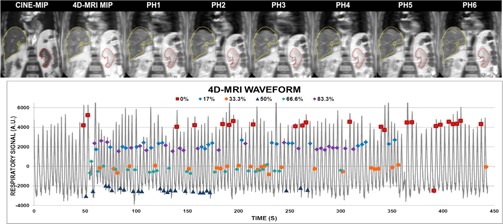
(Top) Coronal view for six‐phase 4D MRI for a liver cancer patient acquired in ~7.4 min. Contours derived from CINE‐MIP. (Bottom) 4D MRI waveform with respiratory tagging shown.
